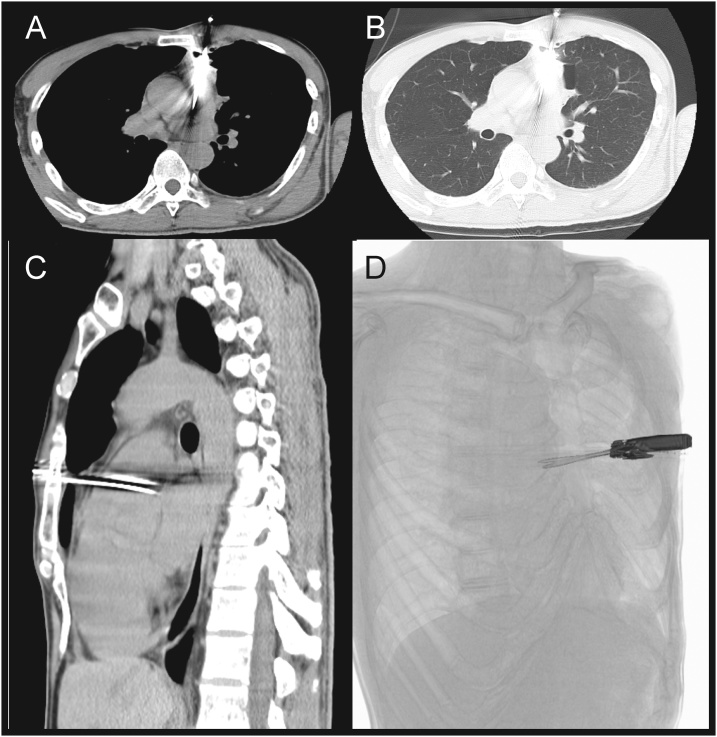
Computed tomography of the chest showed linear metallic density in the pulmonary trunk and a small amount of pericardial fluid.
